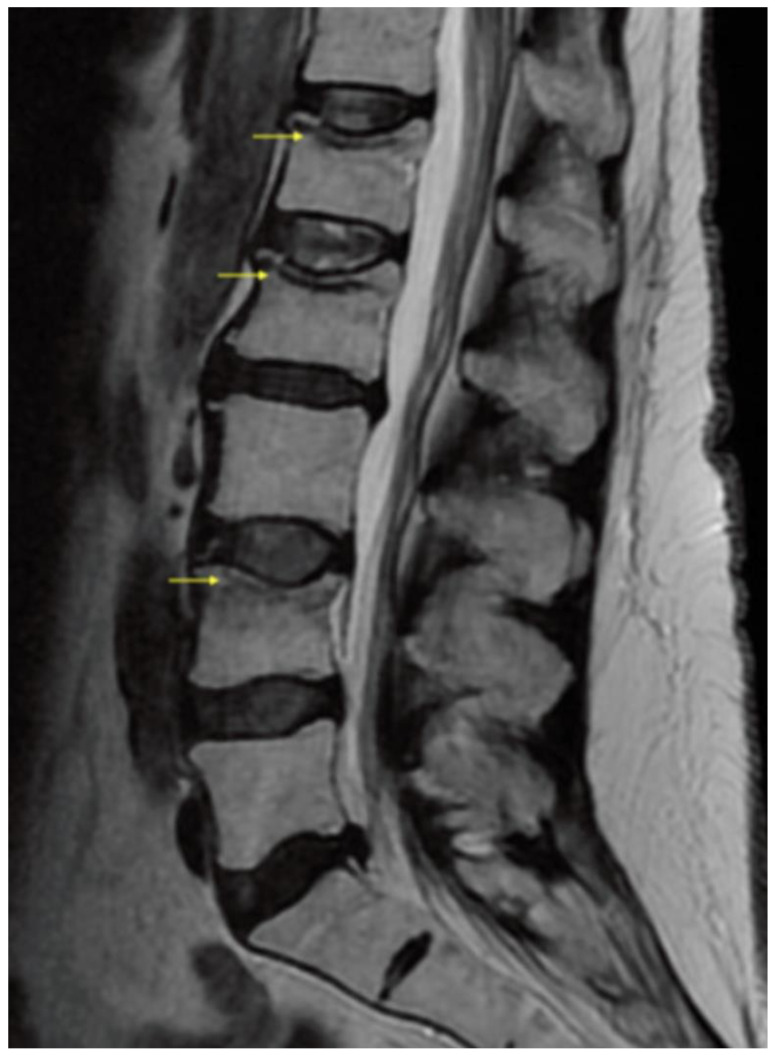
Lumbar spine magnetic resonance T2 image (MRI) with yellow arrows demonstrating superior endplate vertebral compression fractures of the L1, L2 and L4. There is mild postcontrast enhancement at the L1 and L2 compression fractures with minimal STIR hyperintensity edema and moderate postcontrast enhancement involving the superior L4 endplate.
